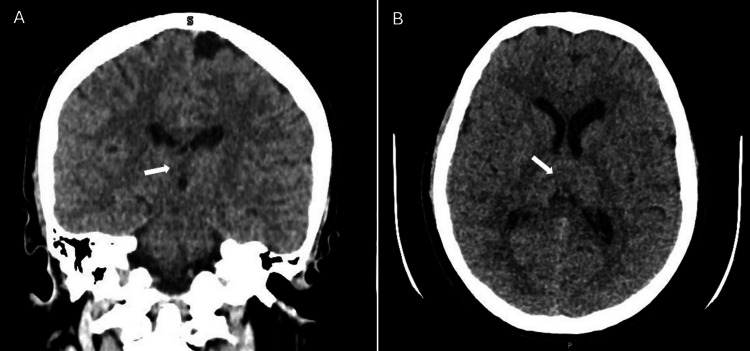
CT images of the patient’s brain. Coronal (A) and transverse (B) views of brain CT showing a hypodense area in the floor of the third ventricle (white arrows).
